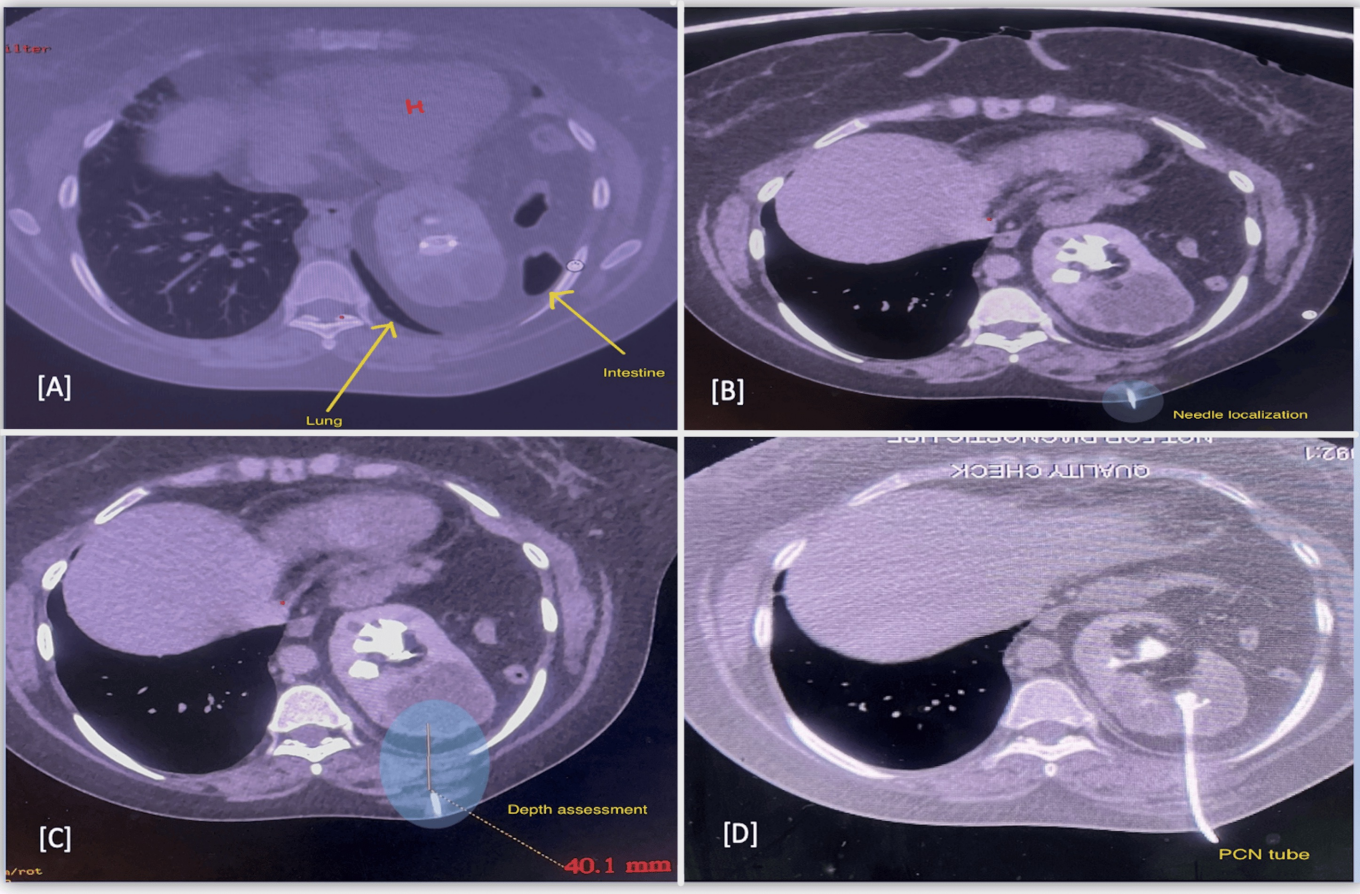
Computed tomography guided left percutaneous nephrostomy placement (axial sections). (A) Narrow window for puncture due to lung on one side and intestine on the other (H: heart); (B) needle localization; (C) depth perception; (D) nephrostomy tube in situ. PCN tube: percutaneous nephrostomy tube.
